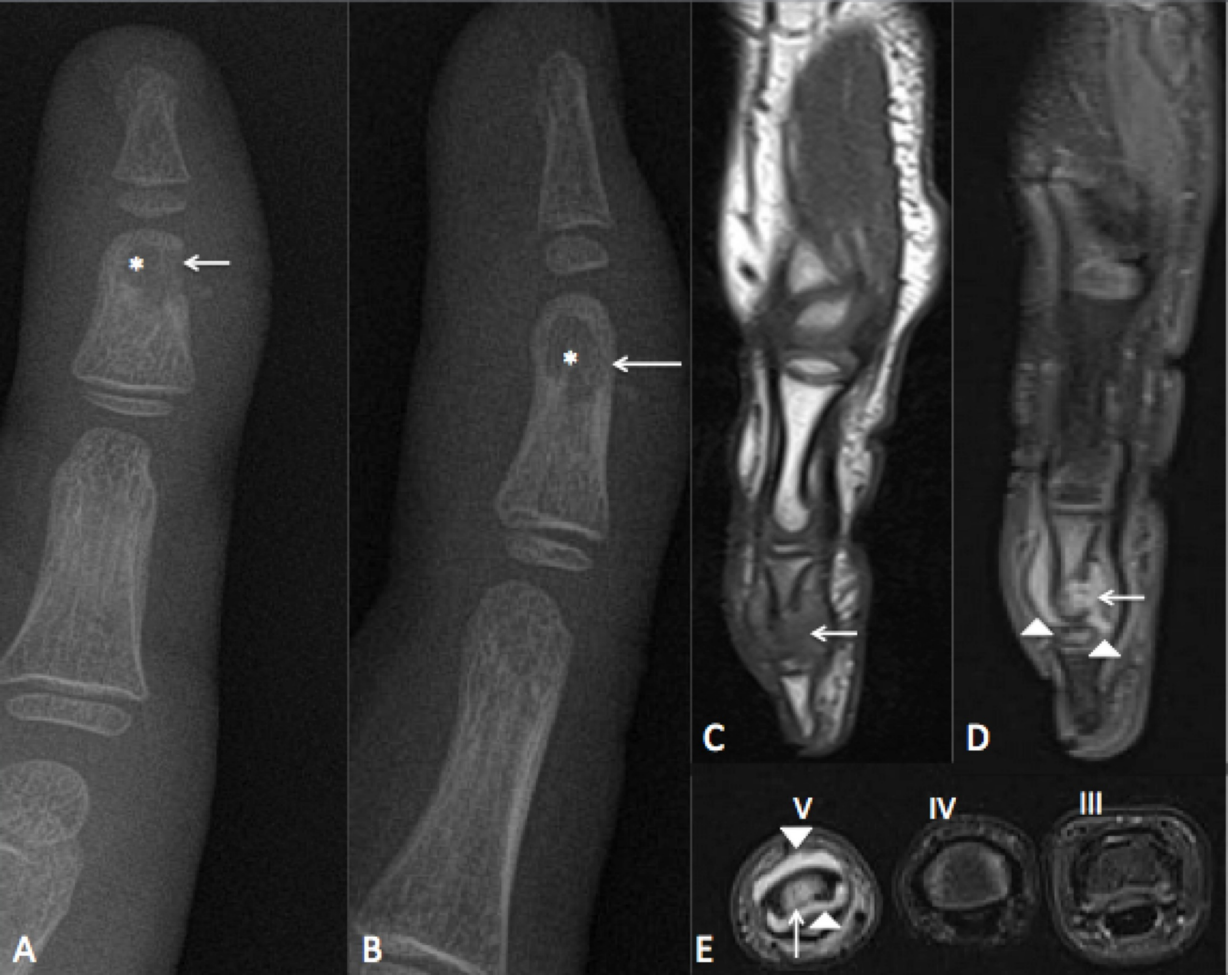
Radiographs and MRI findings. A) Frontal view radiograph of the fifth finger showing a round radiolucent lesion (*) with cortical interruption (arrow) and swelling of surrounding soft tissues; B) Lateral view radiograph showing the same findings; C) Sagittal T1-weighted images showing a low-signal bone marrow replacement lesion with cortical interruption of palmar aspect of finger (arrows); D) Sagittal T2-weighted fat-sat images showing a high signal bone marrow edema due to granulomatous reaction with cortical defect (arrow). The inflammation extended to surrounding soft tissues and to interphalangeal joint (arrowheads); E) T2 weighted fat-sat images on third (III), fourth (IV), and fifth (V) fingers. High-signal bone edema, cortical defect (arrow), and inflammation extension to surrounding soft tissues (arrowheads) can be seen.
